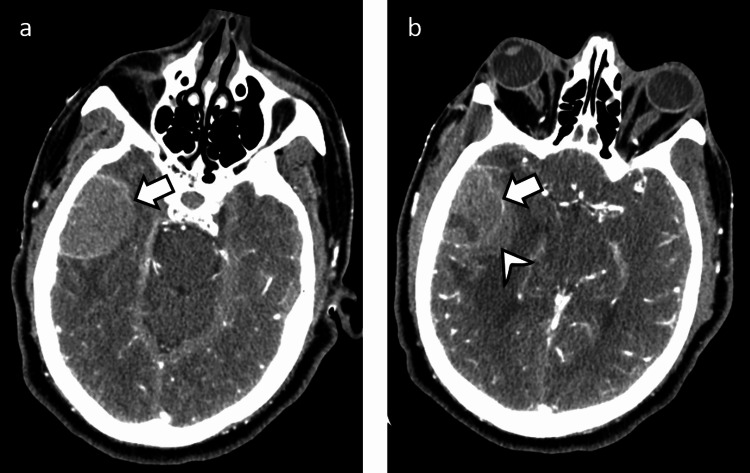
CTA head a: Portions of the right temporal findings appeared very well circumscribed on the CTA head with a possible thin enhancing rim (arrow). b: In other portions, you can see where hemorrhage (arrowhead) has extended beyond the faintly enhancing rim (arrow) CTA: computed tomography angiography
